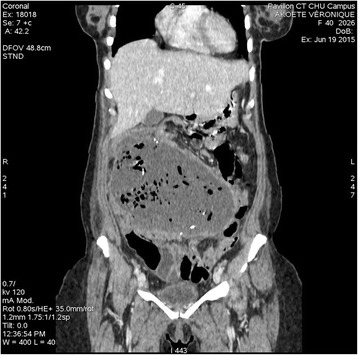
Cornal abdominal CT scan image;large well limited hypodense mass (gossypiboma) withaeric content and rares calcifications
